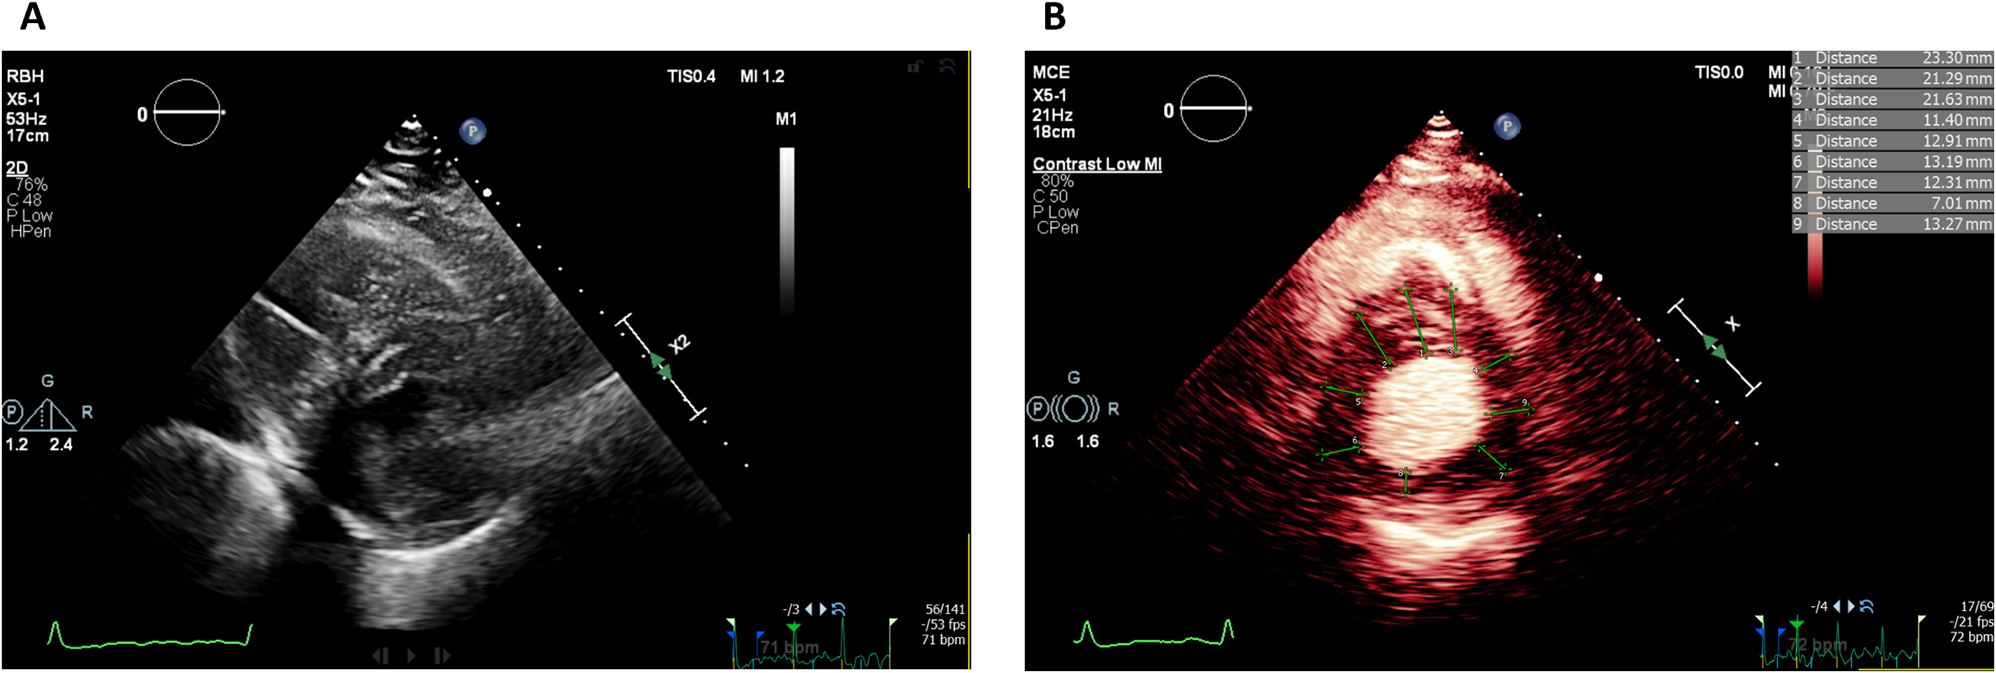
Unenhanced parasternal short axis (A) vs contrast enhanced parasternal short axis (B) at the mid-ventricular level in the same hypertrophic cardiomyopathy patient permitting accurate max wall thickness measurement of 23 mm at the mid-anteroseptal wall at end-diastole
